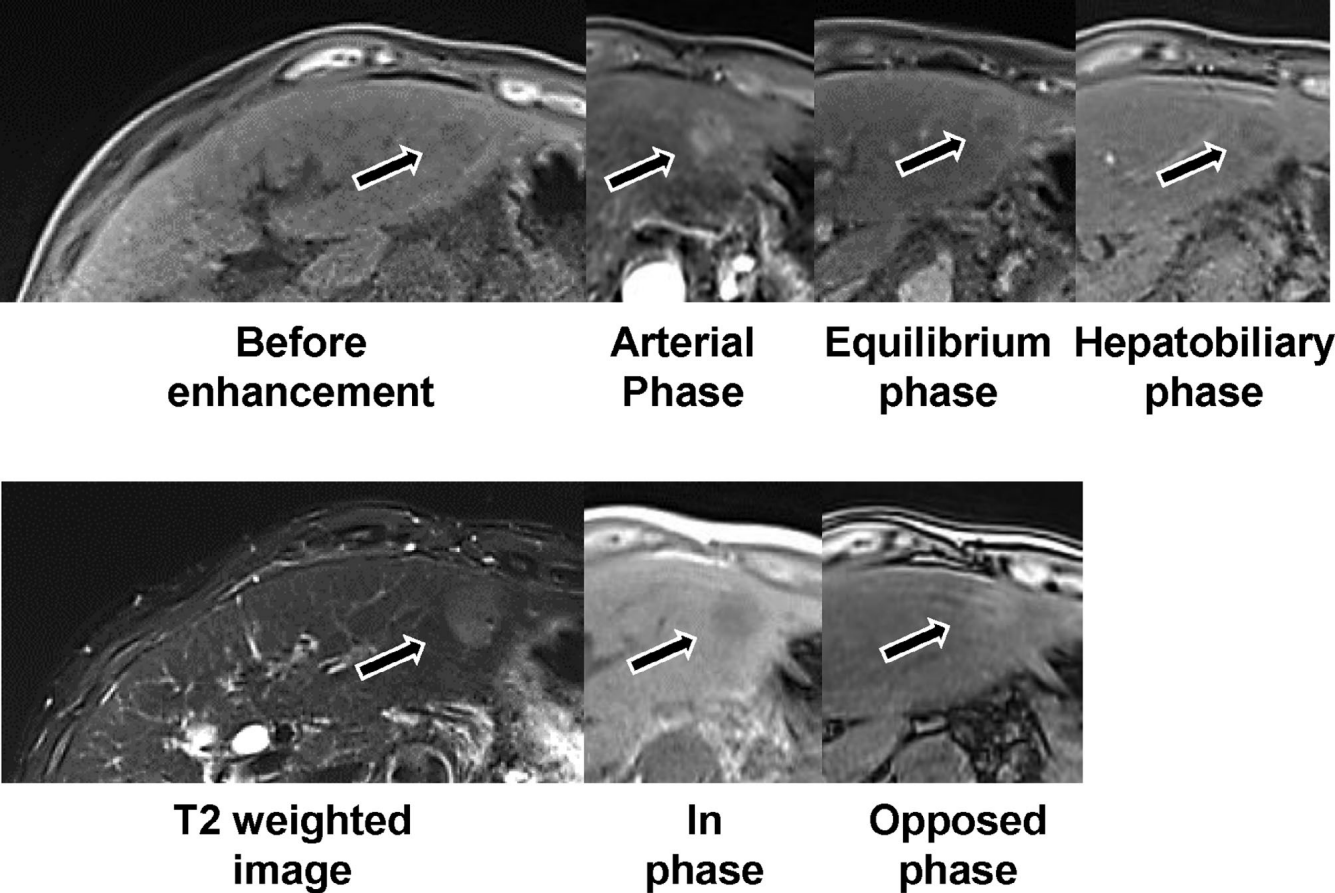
Magnetic resonance imaging (MRI) of the liver. The liver exhibited mildly dull‐edged surface and high‐intensity signal in T1 in‐phase and low in opposed phase in MRI. The tumor revealed low intensity in T1 in‐phase and high in opposed phase, high‐intensity signal in T2‐weighted image, and early enhancement effect in the arterial phase and low intensity in the hepatobiliary phase of Gadolinium‐ethoxybenzyl‐diethylenetriamine pentaacetic acid (Gd‐EOB‐DTPA) MRI (black arrows).
